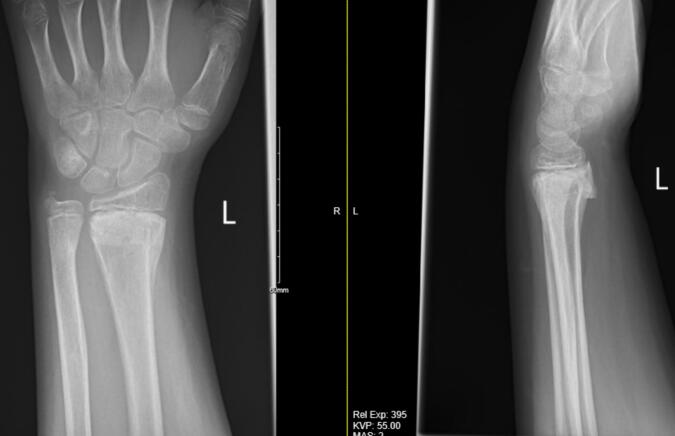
Final radiographs at 8 weeks showing healed radius & ulna fractures.
